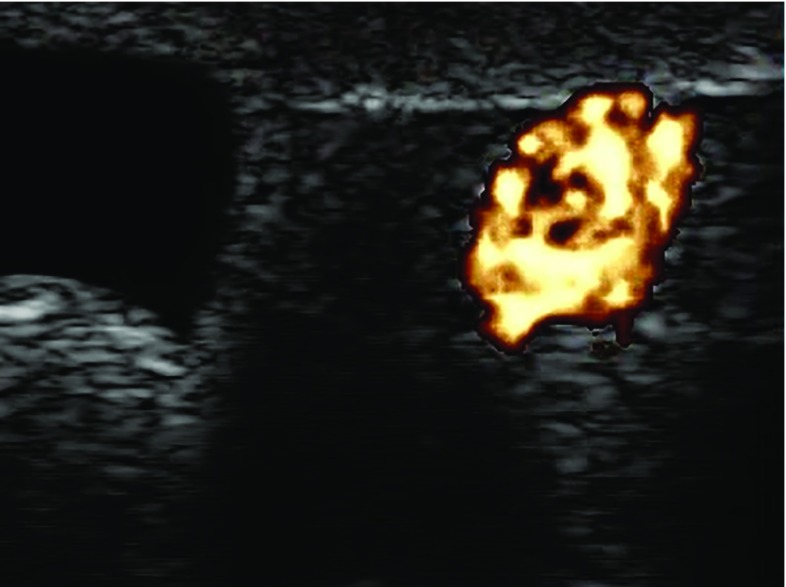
Ultrasound image of a benign cyst model (left) and vessel (right) with flow. Hepatocellular carcinoma tumor model surrounds both hollow structures
